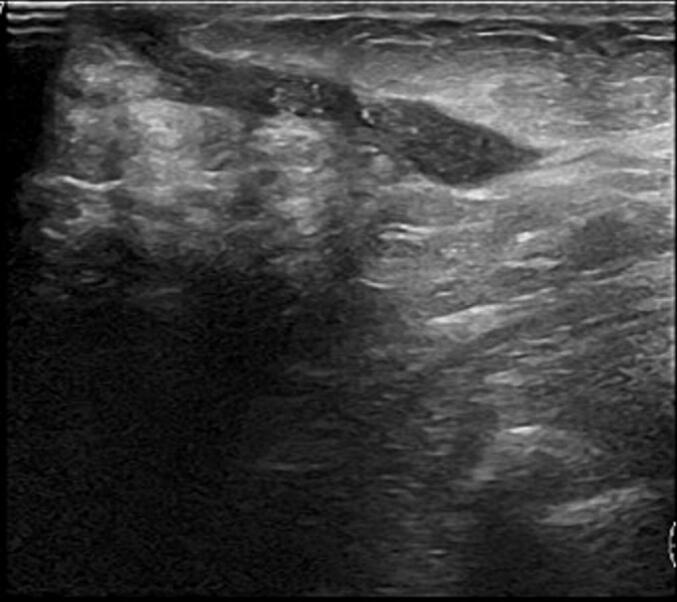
The ultrasound showed abscess coletion and a fistulous tract to the skin.
